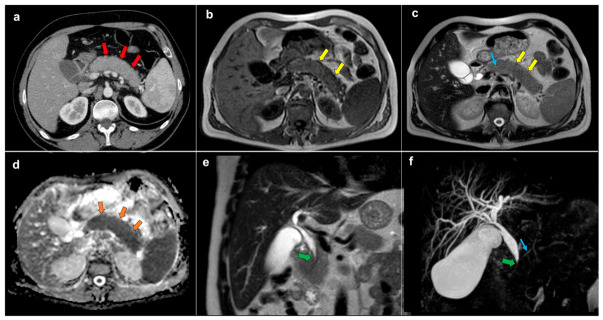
Diffuse AIP in a male patient presenting with painless obstructive jaundice. (a) Axial pancreatic-phase CT shows a “sausage-shaped” pancreas with diffuse enlargement and loss of lobulations (red arrows). (b) Axial T1- and (c) T2-weighted MR images demonstrate a subtle hypointense peripancreatic “halo” (yellow arrows) and a mild diffuse increase in pancreatic T2 signal related to inflammation. Only a short segment of the main pancreatic duct (MPD) is visible on T2WI (blue arrow), due to diffuse stricture caused by fibroinflammatory changes. (d) ADC map shows diffusely low values within the pancreatic parenchyma, consistent with restricted diffusion (orange arrows). (e) Coronal T2WI and (f) 3D MRCP with MIP reconstruction show stenosis and wall thickening of the distal intrapancreatic CBD (green arrow), with moderate upstream biliary dilatation. Note again that only a short segment of the MPD is visible (blue arrow).
